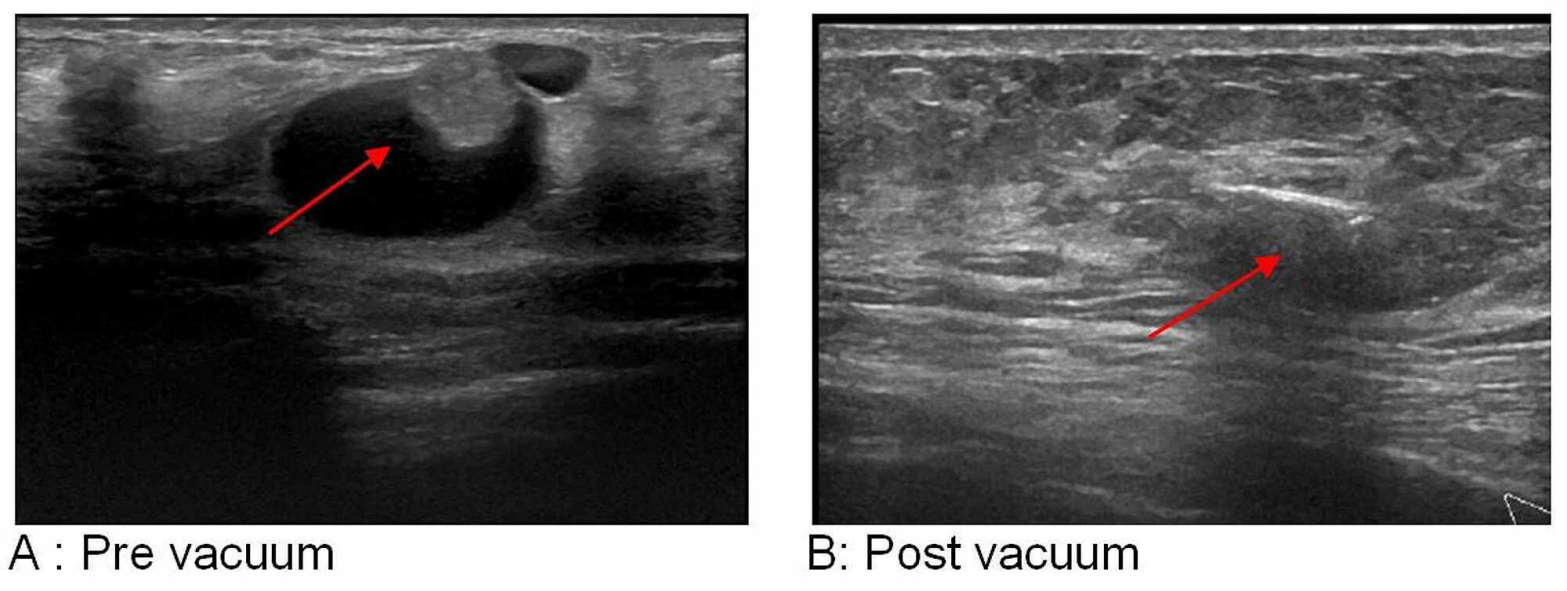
Right breast ultrasound scan of a 10 o’clock-located 18-mm intracystic papilloma in a 44-year-old female patient
